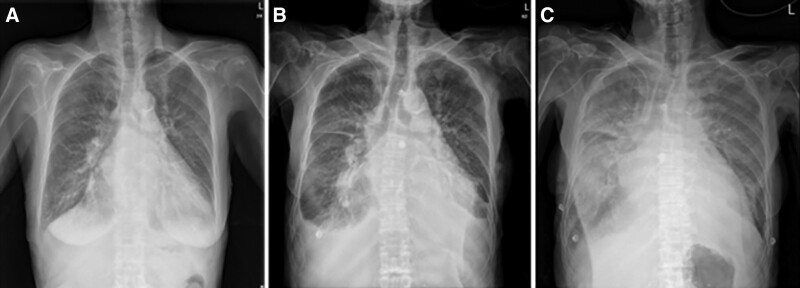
Serial change of chest radiography during the admission period. The chest radiograph demonstrates acute exacerbation of uncontrolled flash pulmonary edema during hospitalization. (A) Day 36 of admission. (B) Day 38 of admission. (C) Day 39 of admission.
